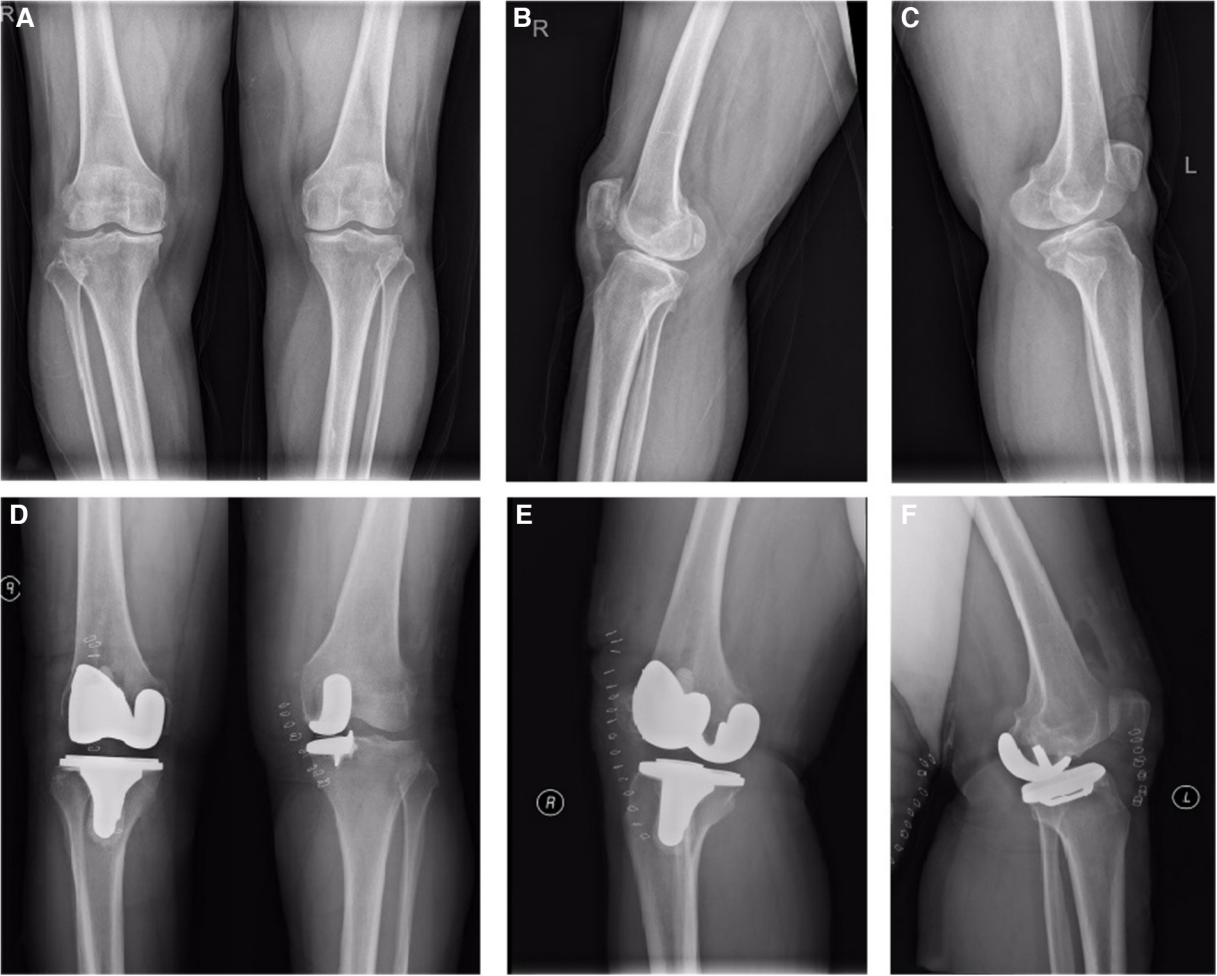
pre-operative (10 days) and post-operative (2 days) X-ray examinations of a 68-year-old women with bilateral osteoarthritis. (A) Anteroposterior position 10 days before operation. (B) Lateral position of right knee joint 10 days before operation. (C) Lateral position of left knee joint 10 days before operation. (D) Anteroposterior position 2 days after operation. (E) Lateral position of right knee joint 2 days after operation. (F) Lateral position of left knee joint 2 days after operation.
